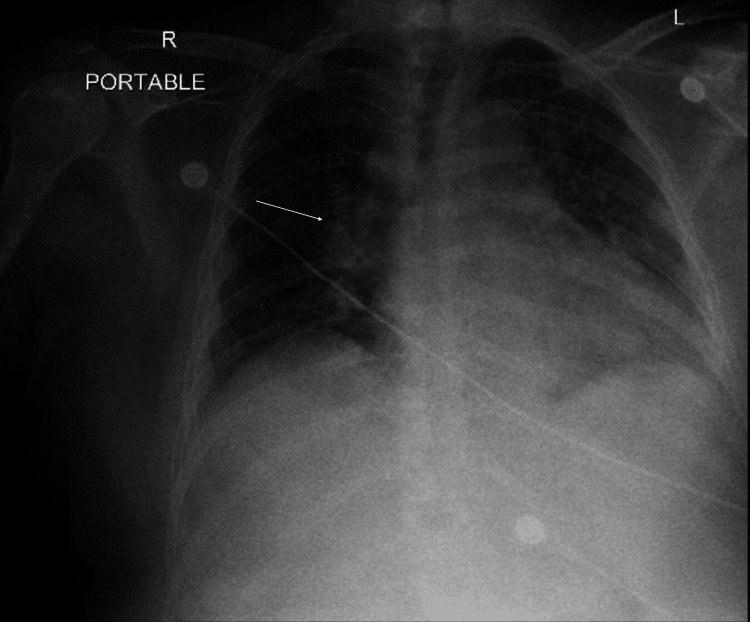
Chest X-ray showing improvement in the status of the pulmonary vasculature (arrow) and clear lung fields three days after the patient’s intubation in the post-anesthesia care unit
